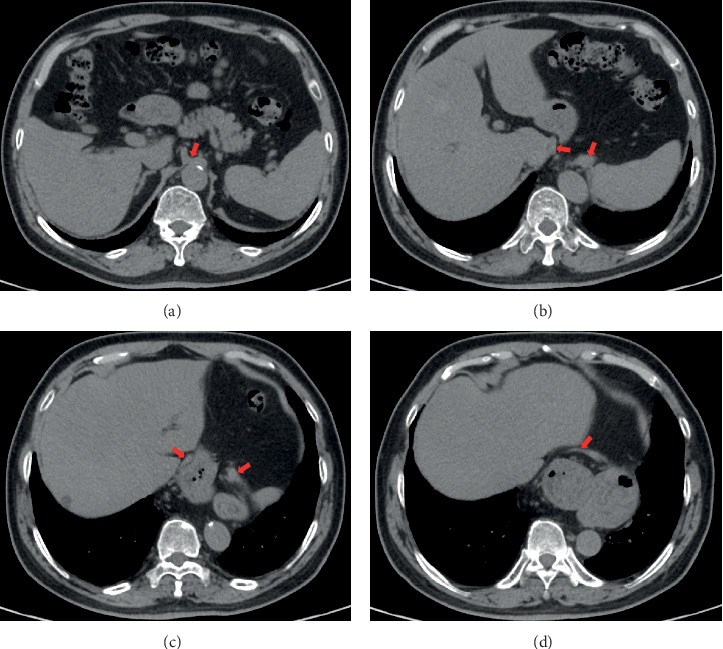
CT images showed the diaphragmatic crura from bottom to top: (a) the red arrow indicated the position where left and right crura began to separate; (b, c) the red arrow indicated the separated crus; (d) the red arrow indicated the left and right crus converge.
